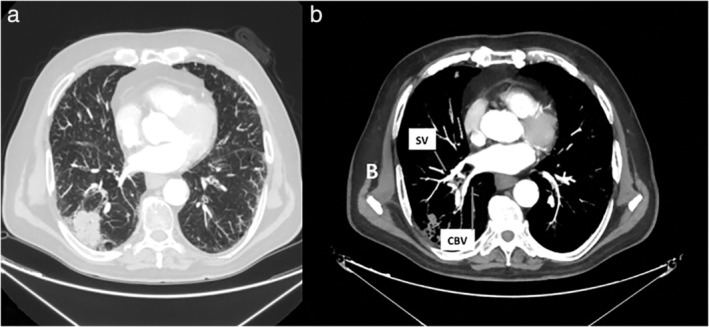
Chest CT scans with maximum intensity projection (MIP) reconstructions. (a) Peripheral solid lesion in the right lower lobe. (b) Superior and common basal veins from the right lower lobe draining separately into the left atrium. SV, superior vein; CBV, common basal vein.
